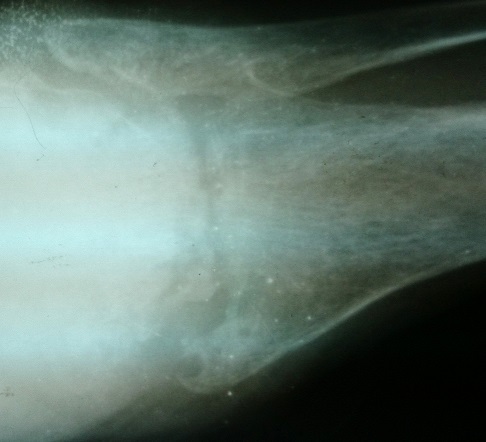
Radiographie de la cheville droite (face) aspect hétérogène de la talo-crurale, pincement de l'interligne, géodes, ostéolyse du dome astragalien et de la malléole interne
